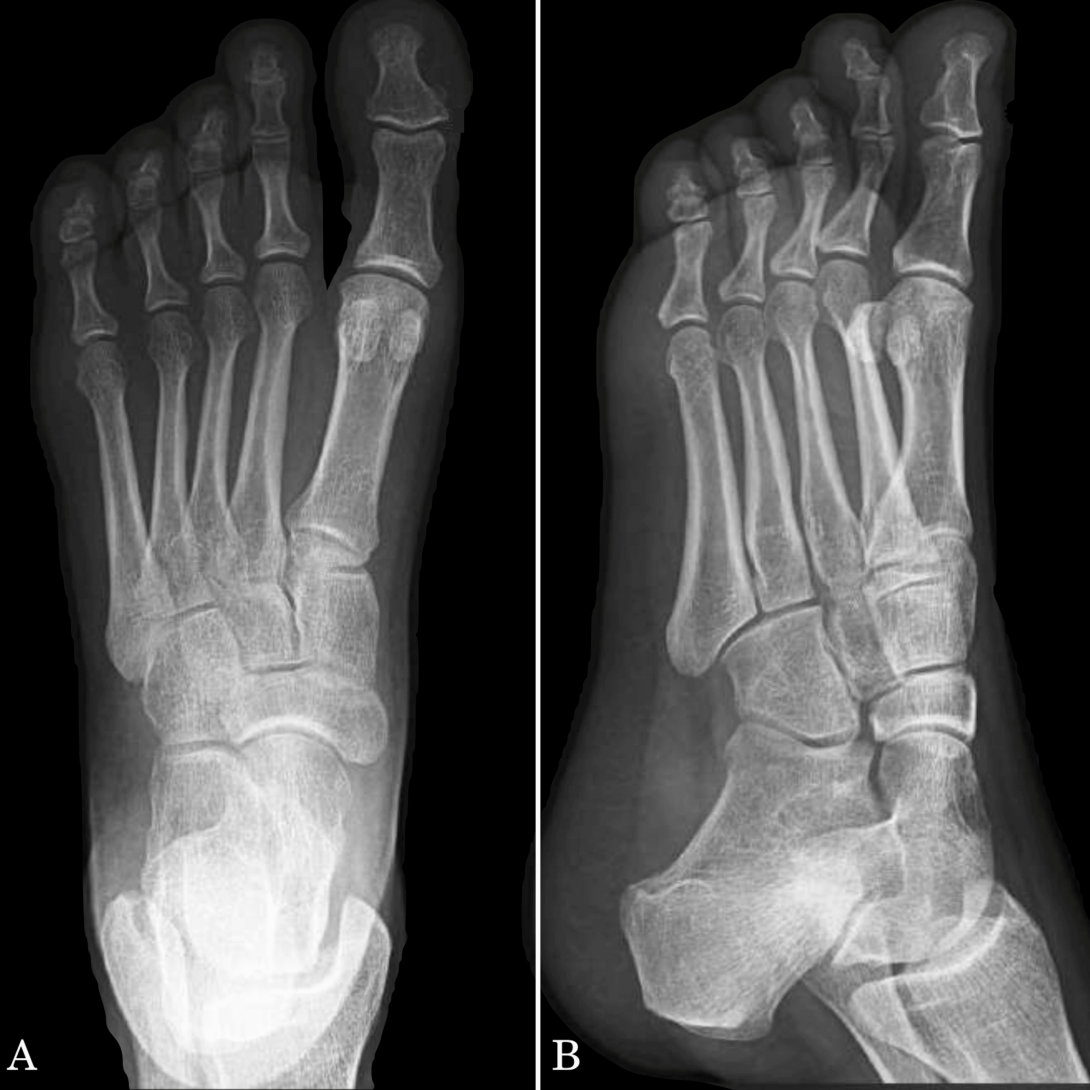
Dorso-plantar and oblique radiograph of the left foot. Normal studies of the left foot of the patient done on the day of the outpatient clinic visit. A: dorso-plantar view; B: oblique view
